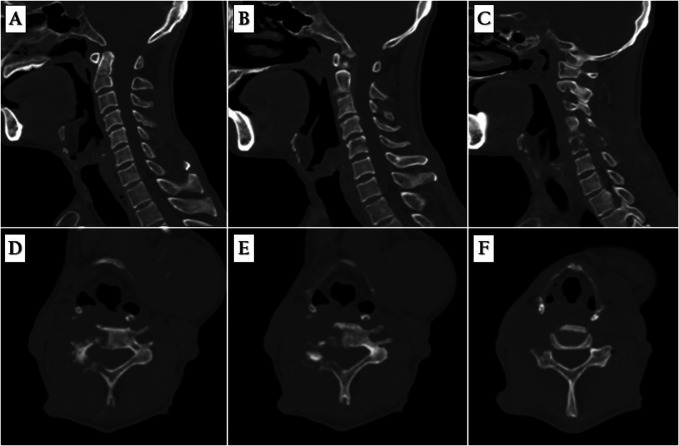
A, Mid-sagittal and (B) left parasagittal CT scan shows no vertebral destruction. C, Right parasagittal CT scan shows osteolytic lesion at C5 vertebral body. D and E, Axial CT scan shows the osteolytic lesion right transverse process and right pedicel at the C5 level and (F) without abnormal lesion at the C6 level.
